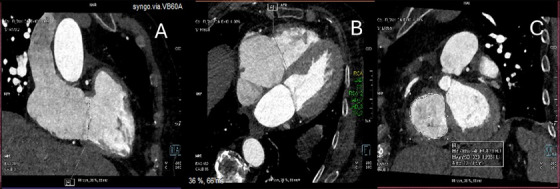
Anulus measurement in CT with multi plane reconstruction. (A) antero‐superior diameter; (B) septo‐lateral diameter; (C) multiplane reconstruction with measurement of the entire anulus. CT indicates computed tomography.
